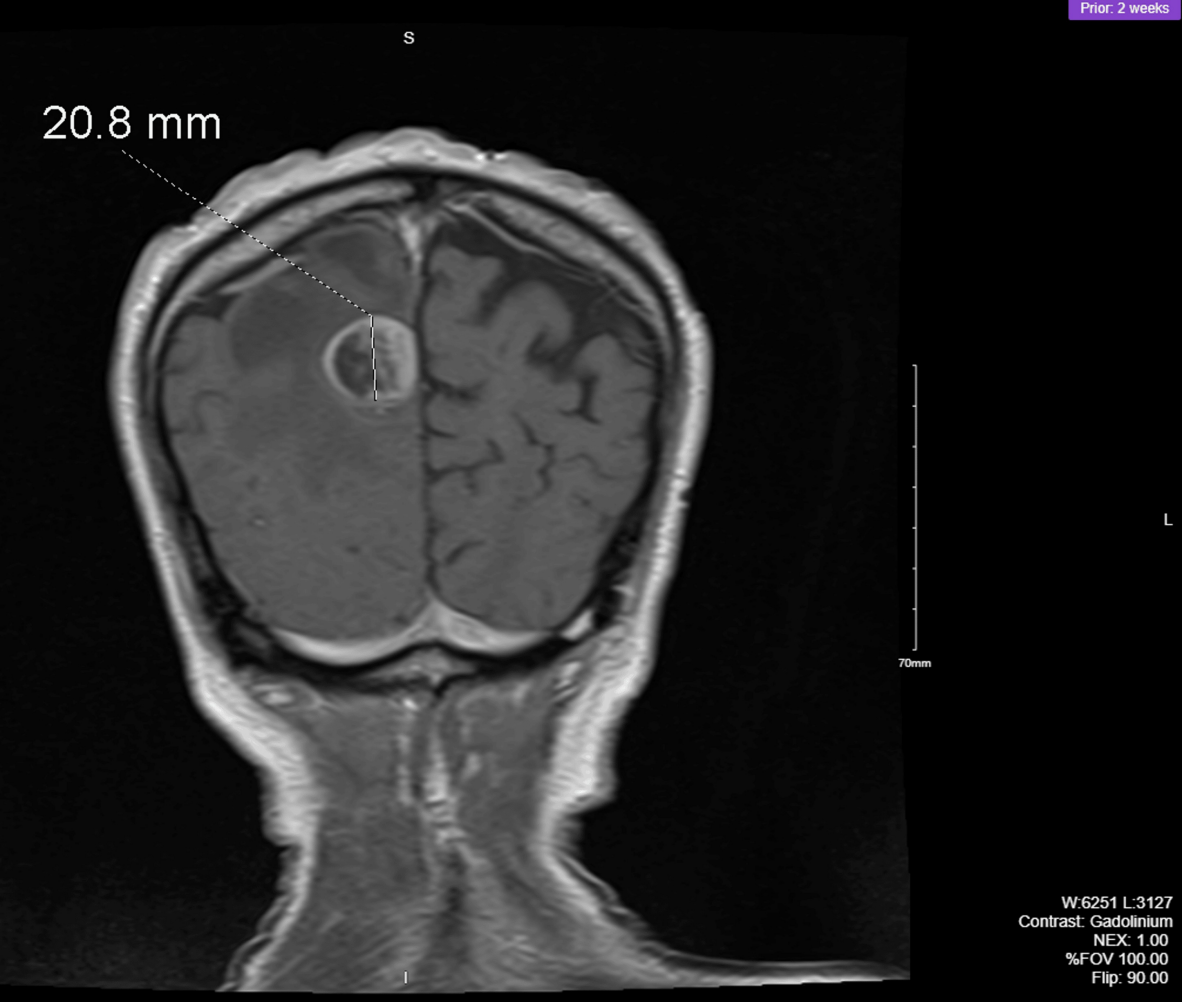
Preoperative brain MRI sagittal view MRI: magnetic resonance imaging
